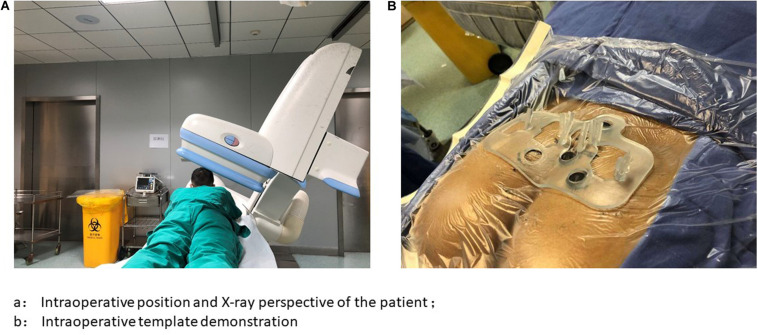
Presentation of X-ray perspective and surgical template. (A) Intraoperative position and X-ray perspective of the patient; (B) Intraoperative template demonstration.
